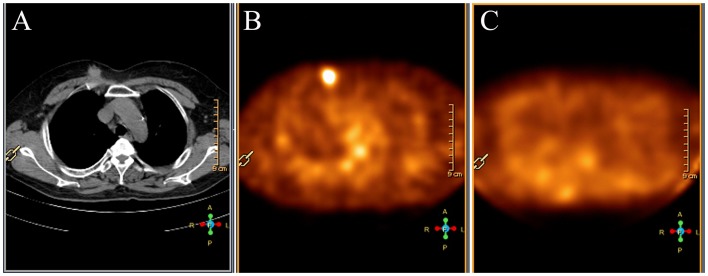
A 2.2 cm ductal carcinoma in situ of the right breast in a 31-year-old woman (Patient 15). (A) CT scan demonstrates a mass in the right breast. (B) ^99m^Tc-3P_4_-RGD_2_ SMM demonstrates focal uptake of ^99m^Tc-3P_4_-RGD_2_ in the tumor (T/NT = 2.96). (C) ^99m^Tc-MIBI SMM demonstrates low uptake of ^99m^Tc-MIBI in the tumor (T/NT = 1.31).
